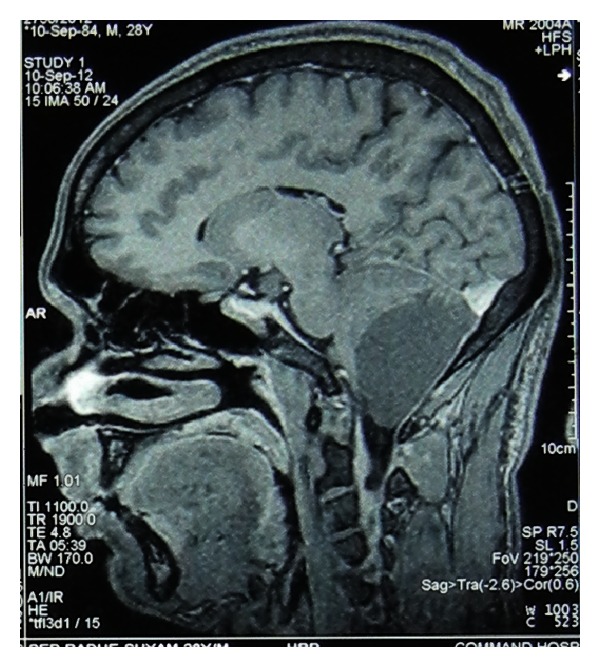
MRI (sagittal view) Showing mass in posterior fossa.
